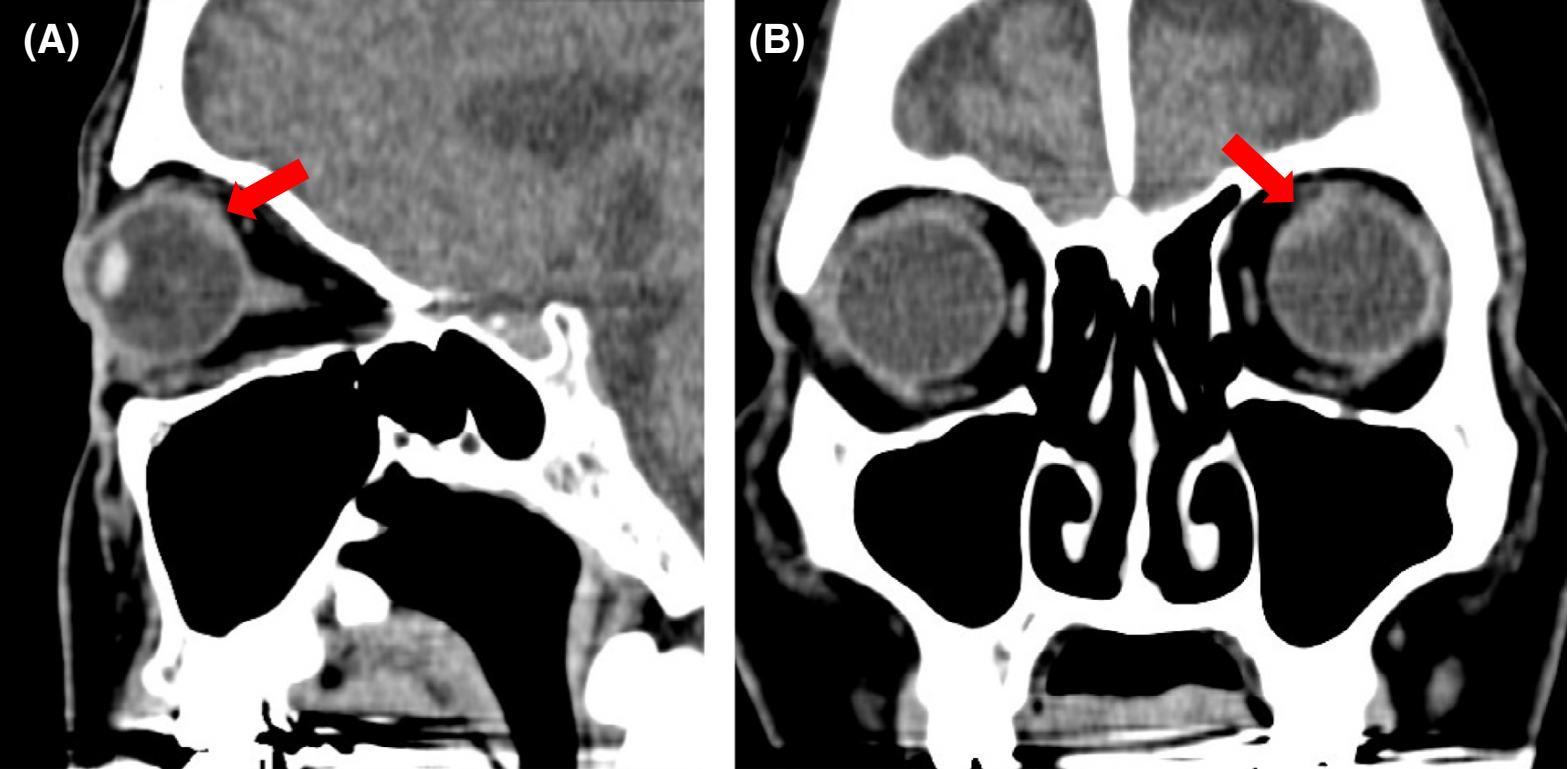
(A, B) Computed tomography showing thickness at the inner upper side of the posterior pole of the left eye.
